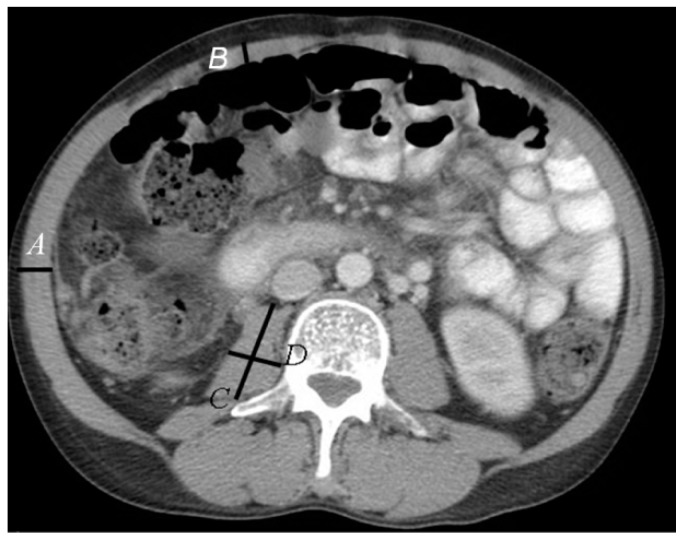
Assessment of muscle wasting at the third lumbar vertebra (L-3). Cross-sectional psoas muscle area measured using computed tomography imaging (CT) at the level of the third lumbar vertebra: A: lateral wall muscle measurement; B: abdominal wall muscle 2 cm to the right of the midline; C: anterior/posterior length measurement; D: transverse psoas diameter.
